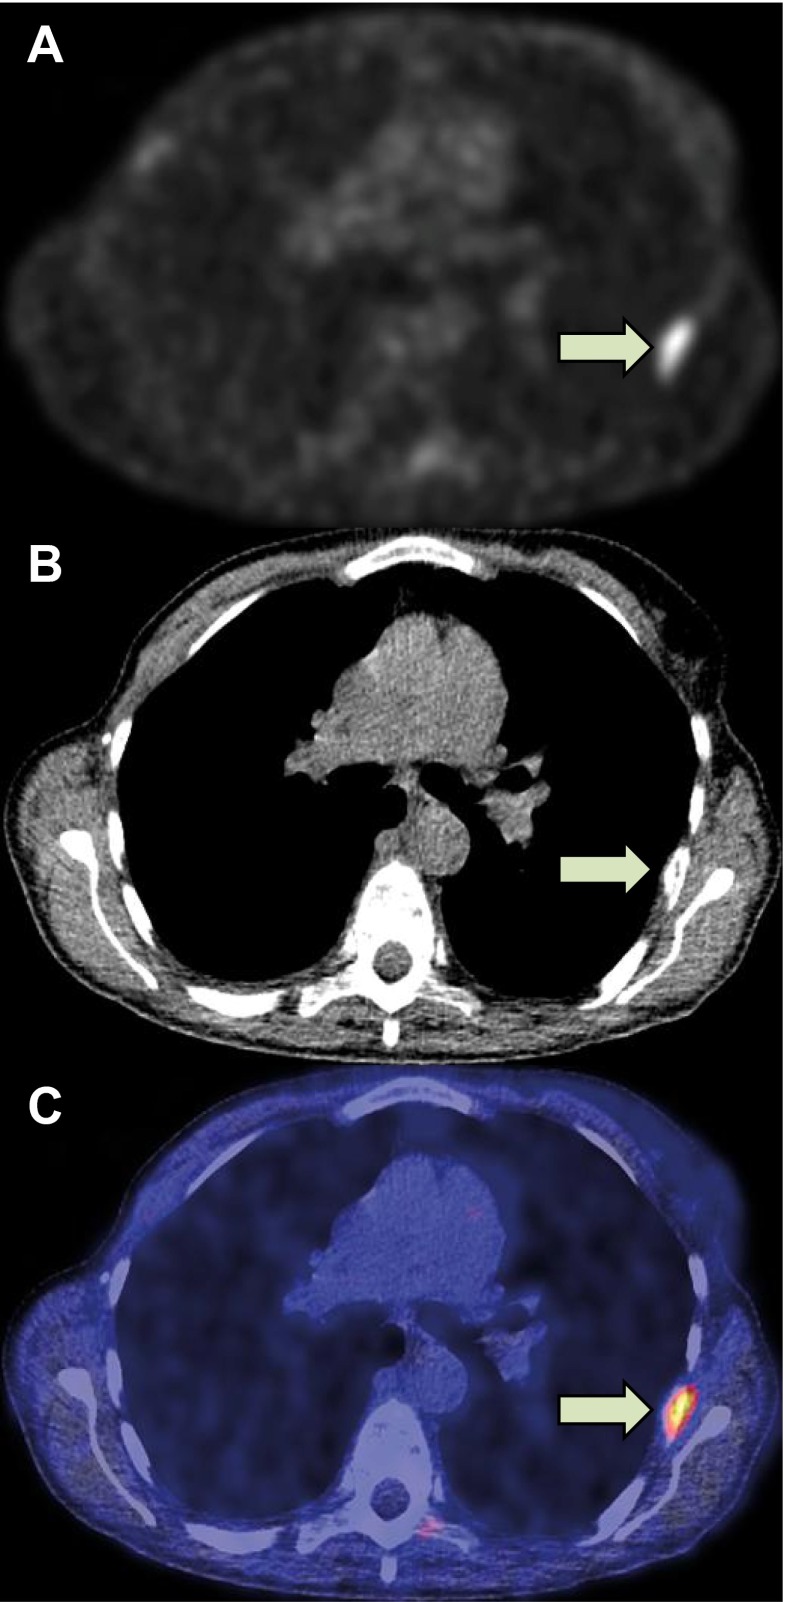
^18^F-FES-PET (a), CT (b) and ^18^F-FES-PET/CT (c) of a patient with bone metastases. Indicated is a rib metastasis (*arrow*) with SUV_max_ 3.3
